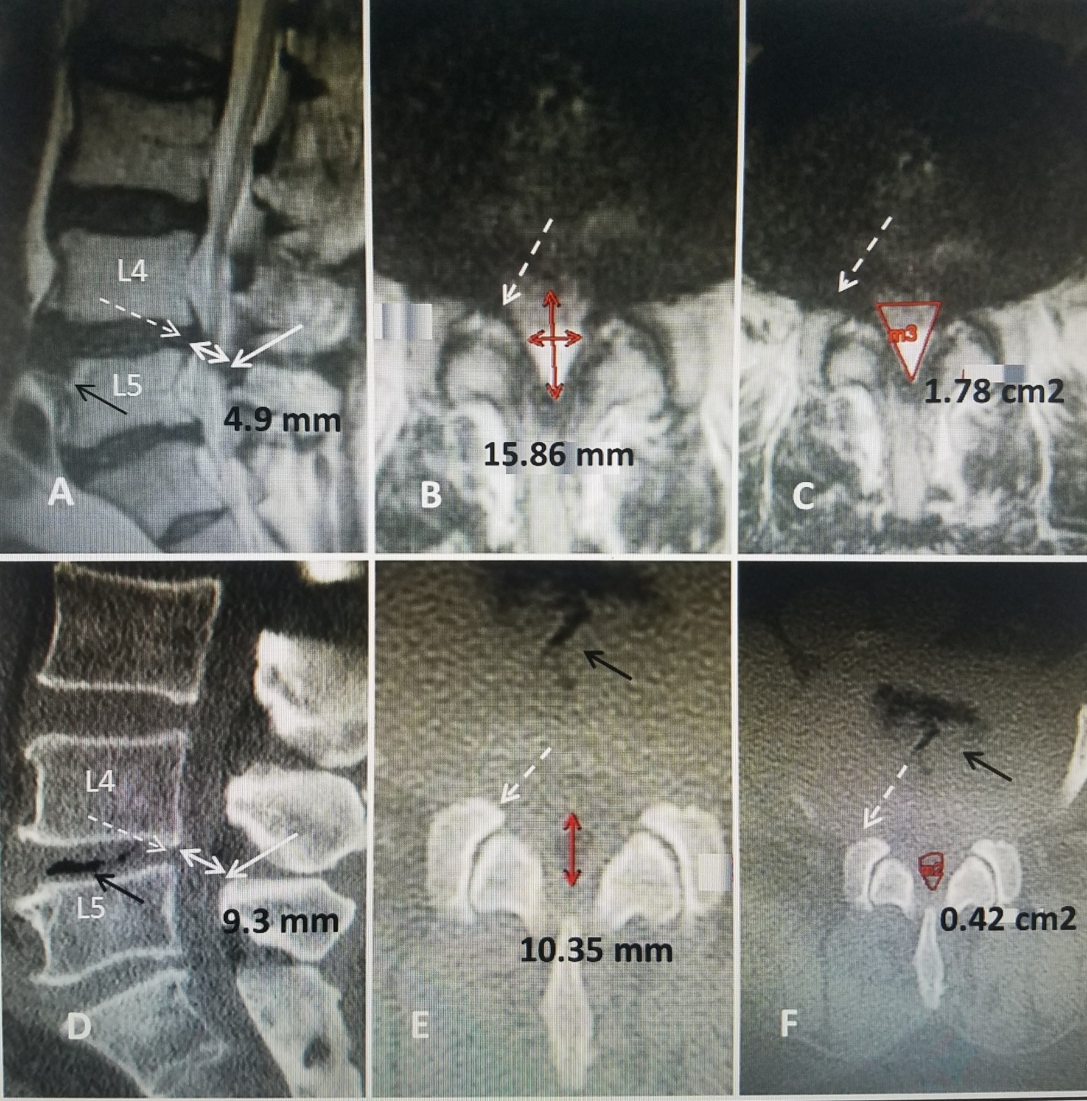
Lumbar canal measurements comparing both MRI and CT scans in the same planes in the same patient with L4-5 stenosis. A: Sagittal T2 MRI showing L4-5 compression from combination of a ventral herniated disc (dashed white line) and posterior ligamentous compression (solid white arrow). The anterior-posterior canal dimension is 4.9 mm (double-headed white arrow). B; Axial T2 MRI shows a midline sagittal measurement of 15.86 mm. The mid-facet line measurement is 7.22 mm (horizontal solid red arrow). The lateral disc that is seen in 'A' (dashed white arrow). C: Calculating axial area using an area cursor gives canal area of 1.78 cm^2^. The lateral disc is seen at L4-5 (dashed white arrow). D: Sagittal CT scan performed on same patient. The anterior-posterior canal dimension is 9.3 mm (solid white double-headed arrow). The midline sagittal slice is similar to the MRI scan in 'A'. The anterior-posterior canal dimension is 9.3 mm at the L4-5 level. Vacuum changes can be seen within the L4-5 disc space (solid black arrow). Vacuum changes are considered a secondary radiologic sign for both disc degeneration and possibly segmental instability. The difference in anterior-posterior canal dimension from CT to MRI is 4.4 mm, indicating significant soft tissue narrowing. E: Axial CT showing midline soft tissue measurement of 10.35 mm (vertical solid red arrow). There are clear vacuum changes in the disc space (solid black arrow) and lateral foraminal disc compression (dashed white arrow). F: Axial CT measuring the approximate dural area of 0.42 cm^2^ (solid circular red line). This is 24% of the area measured on axial MRI again indicating significant soft tissue rather than bony canal stenosis.
